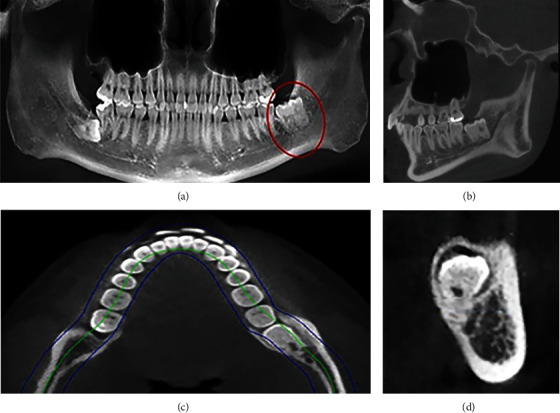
Preoperative CBCT examination. (a) A fourth molar in the mandible left third molar distal; (b–d) the relationship between roots and mandibular canal in coronal, transversal, and sagittal planes.
